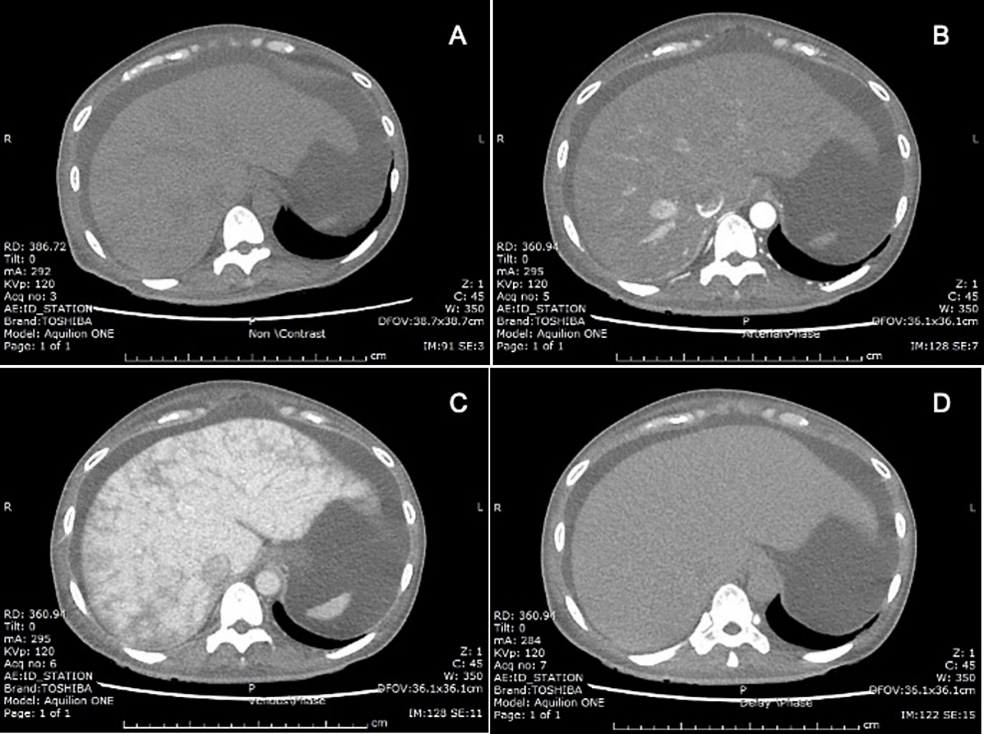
Triphasic abdominal CT scan (A) Non-contrast, (B) arterial phase, (C) portal venous phase, and (D) delayed phase. Hepatomegaly and heterogenous, mottled liver parenchyma with areas of poor enhancement on portal venous phase without demonstrable peritoneal nodule or intra-abdominal lymph node enlargement.
